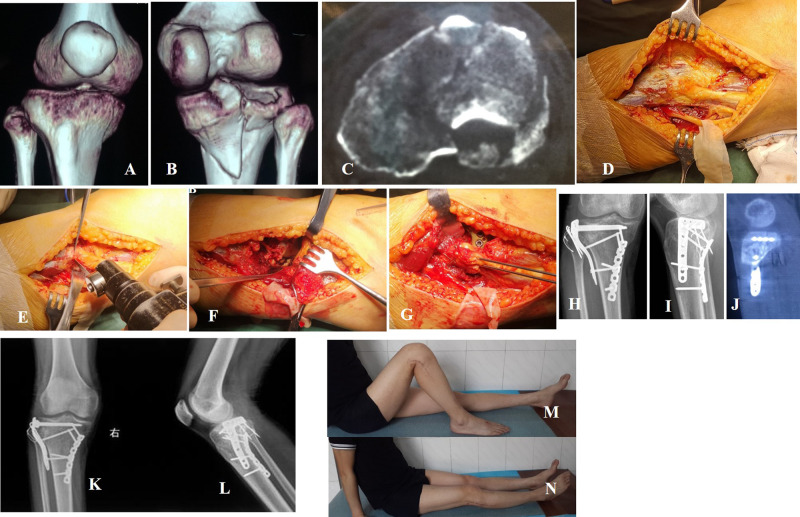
A 26-year-old male patient suffered a posterolateral fracture (Schatzker V) caused by falling from a height. (A–C) Preoperative CT scan and reconstruction confirmed fracture type and obvious displacement. (D) The common peroneal nerve was carefully identified and protected. (E) ‘V’ shape osteotomy of the partial fibular head. (F) Posterolateral articular surface under direct observation. (G) The fibular osteotomy was fixed with a K-wire tension band. (H–J) X-ray and CT scans show an anatomical reduction, good internal fixation, and recovery of the smooth articular surface. (K,L) The fracture healed with no internal fixation failure 1 year after surgery. (M,N) Knee function was excellent at 1 year after surgery.
